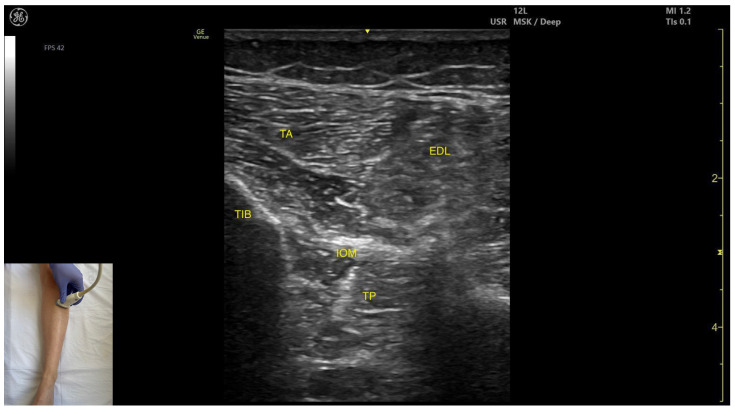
Ultrasound anatomy of the tibialis anterior: TIB—tibia; TA—tibialis anterior; IOM—interosseous membrane; TP—tibialis posterior; EDL—extensor digitorum longus.
